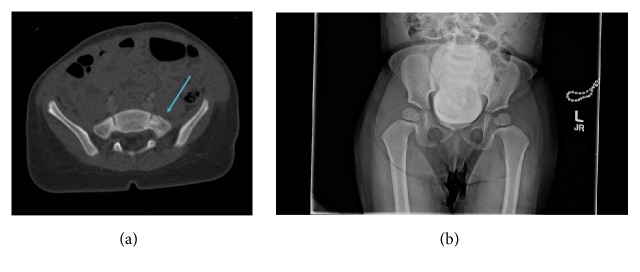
A 2-year-old female presented as a Level 1 Trauma; a contrast-enhanced CT of the abdomen and pelvis (a) was performed, revealing a left sacral fracture (arrow). Subsequently, a radiograph of the pelvis (b) did not demonstrate the same finding, secondary to marked bladder distention following contrast administration for the CT.
